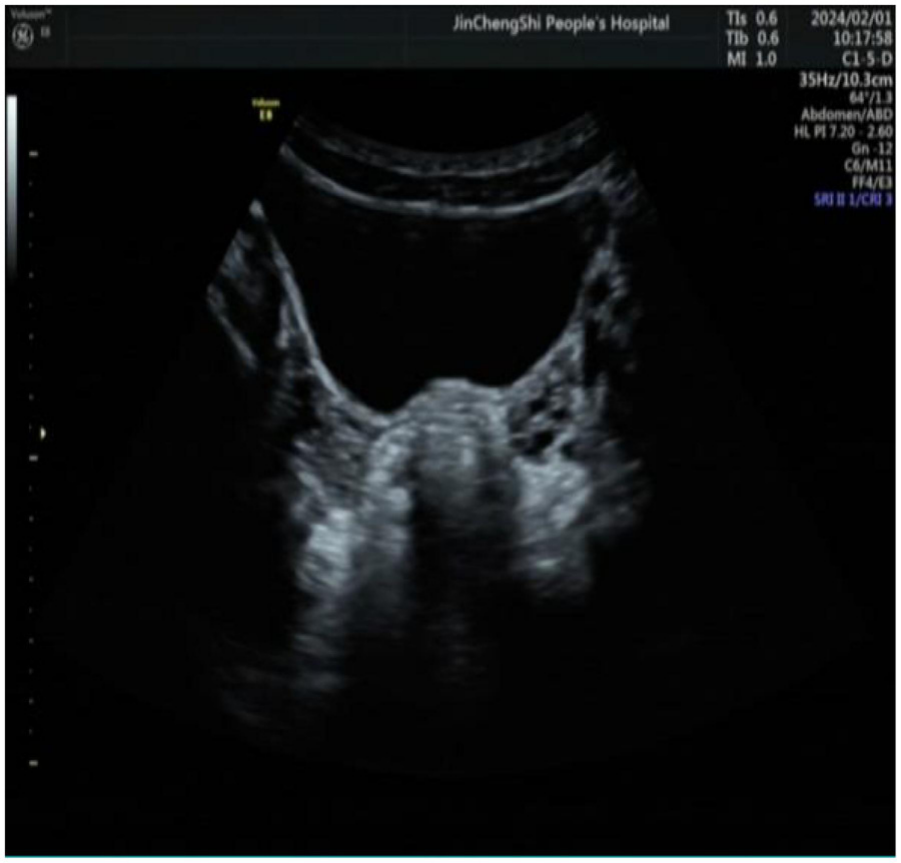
Ultrasound of the patient's uterus and bilateral adnexa.
